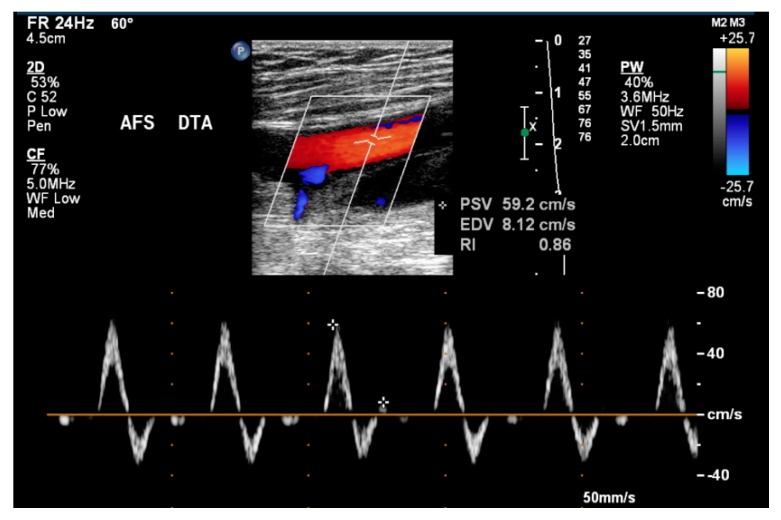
Measurement of systolic and diastolic flow velocities in (cm/s) of the right common femoral artery by lower limb arterial doppler ultrasound.
